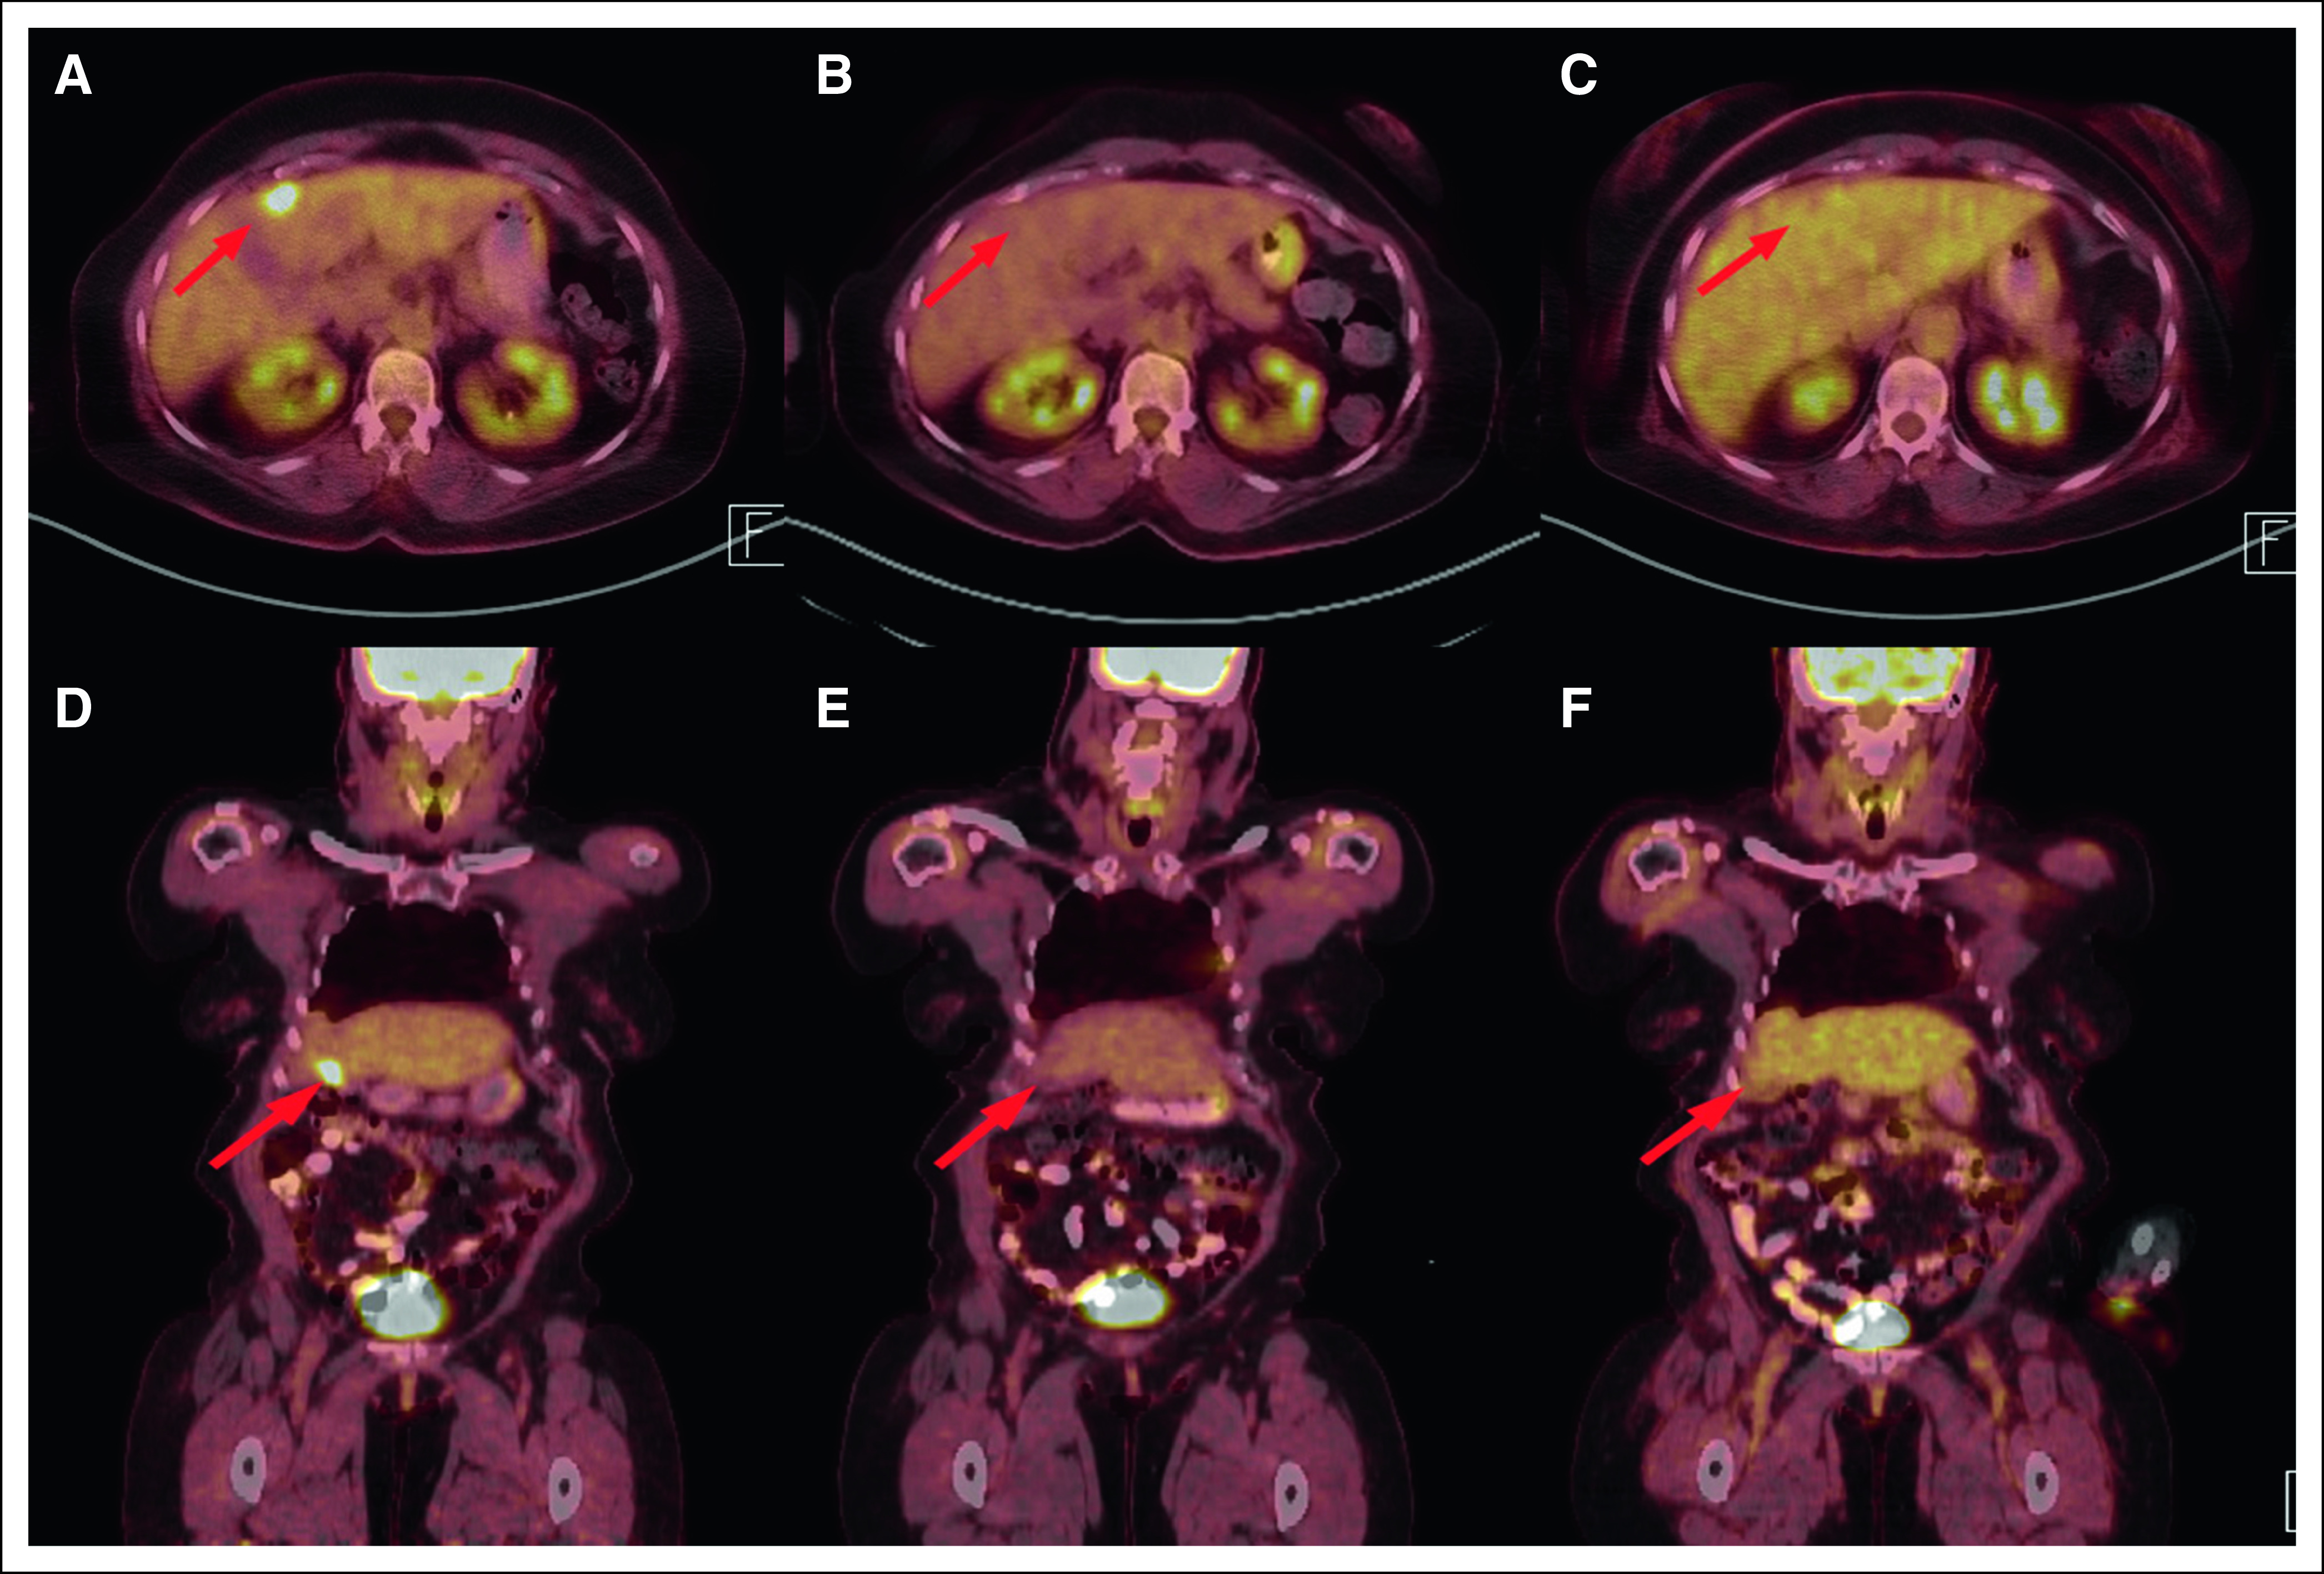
Liver metastasis response to entrectinib. Axial and coronal images at (A, D) baseline, (B, E) 4 weeks, and (C, F) 6 months. The liver metastasis (red arrow) was not well visualized on computed tomography images alone but was [^18^F]fluorodeoxyglucose avid at baseline (standardized uptake value, 12.02) but was not visualized at 4 weeks and remains absent.
